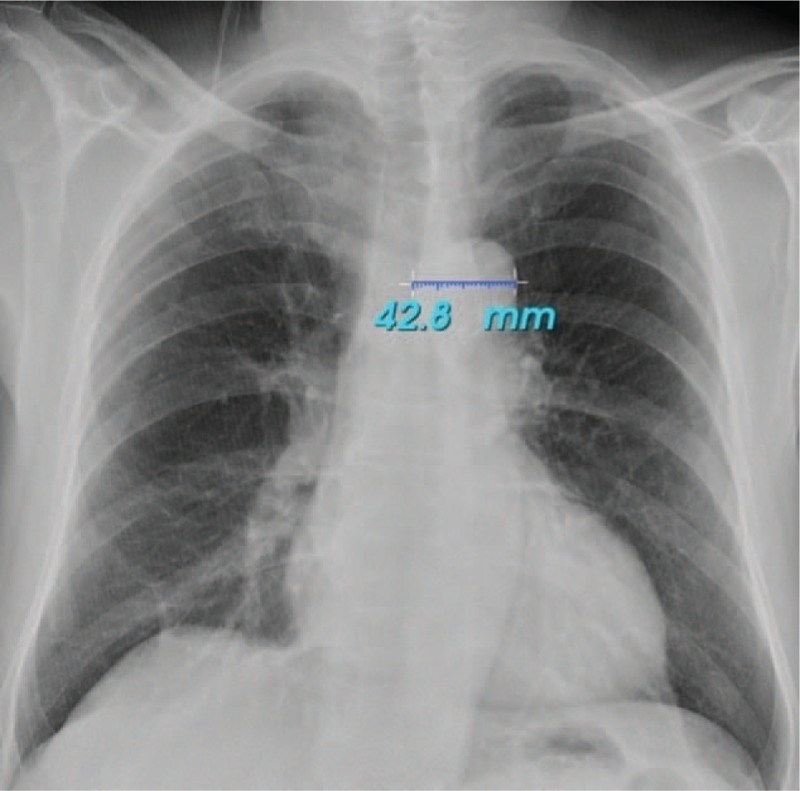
AKW measurement method in chest radiography.
